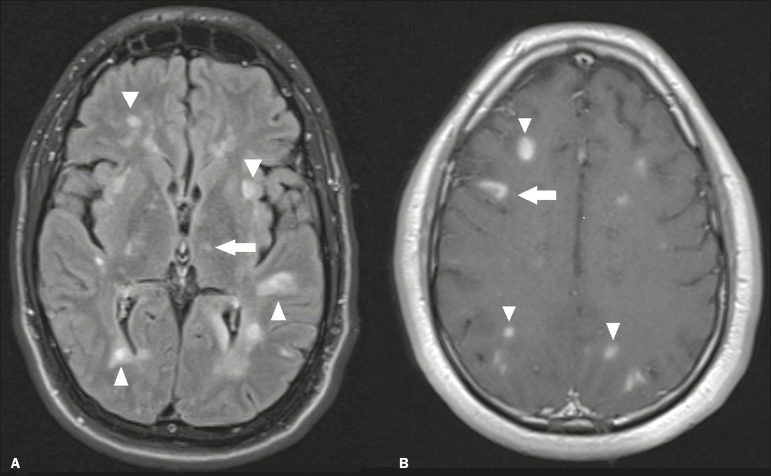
Acute disseminated encephalomyelitis after viral infection. A: Axial FLAIR MRI sequence showing multiple hyperintense lesions throughout the white matter on both sides (arrowheads) and slightly affecting the left thalamus (arrow). B: Contrast-enhanced axial T1-weighted MRI sequence, acquired at a higher level, showing nodular enhancement of some lesions (arrowheads) and discontinuous annular enhancement (arrow) characteristic of demyelinating lesions.
